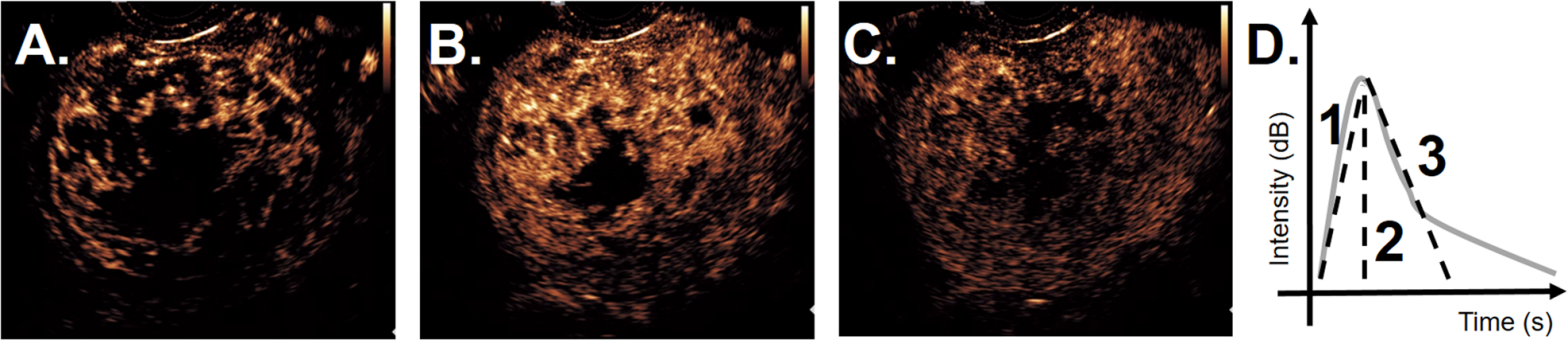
Contrast-enhanced ultrasound images of patient 1 at different time points after injection of contrast agents showing fibroid perfusion, t = 28 s (A); t = 32 s (B); t = 100 s (C). Quantification of the contrast signal results in a time-intensity curve (D), from which parameters such as wash-in rate (1), peak intensity (2) and wash-out rate (3) can be obtained
